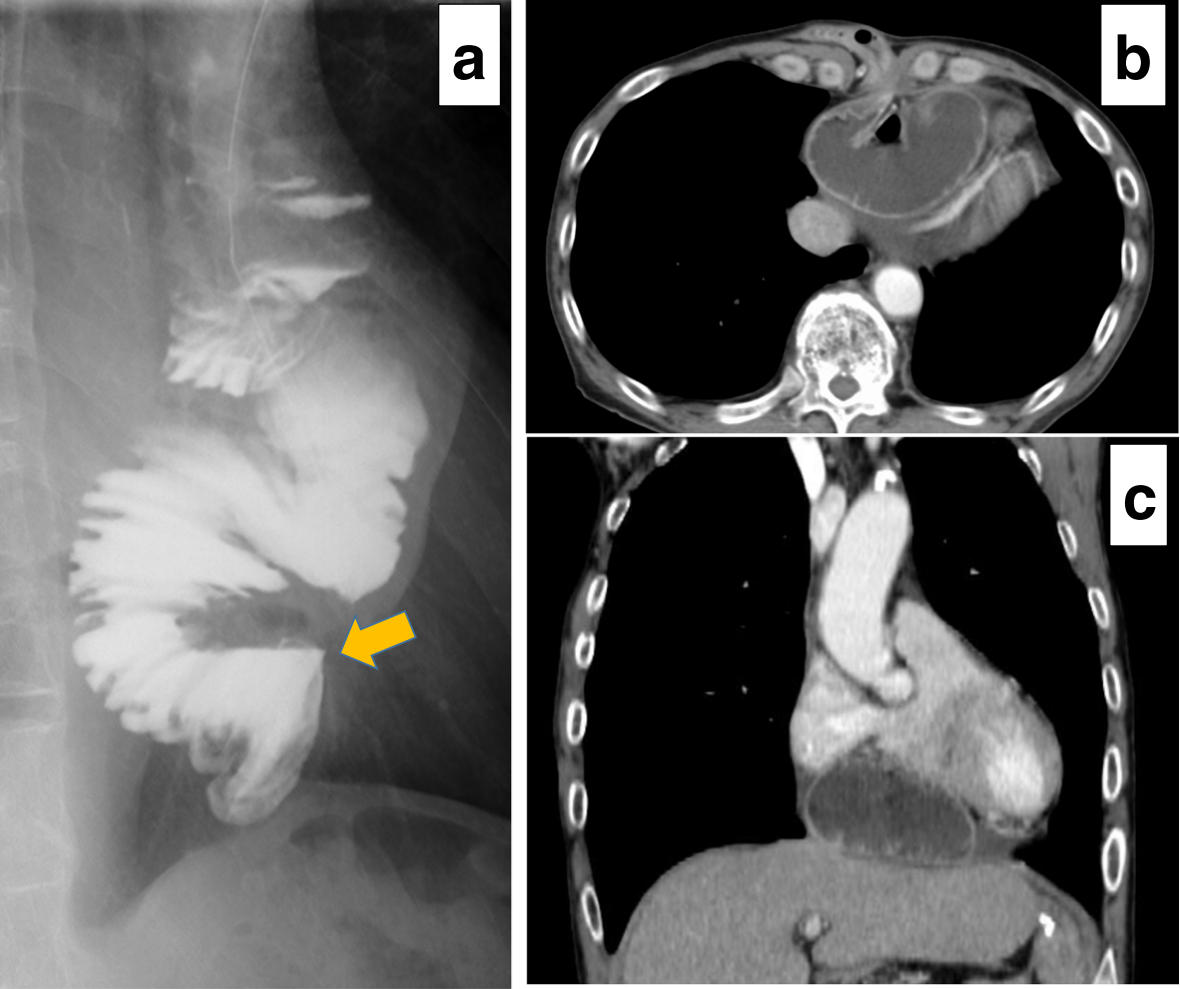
a The pedicled jejunum through the antethoracic route shows the beak sign (arrow) in the enteral contrast study. b Transverse and c sagittal enhanced computed tomography images demonstrate the intrapericardial migration of the small bowel anterior to the heart (arrows)
